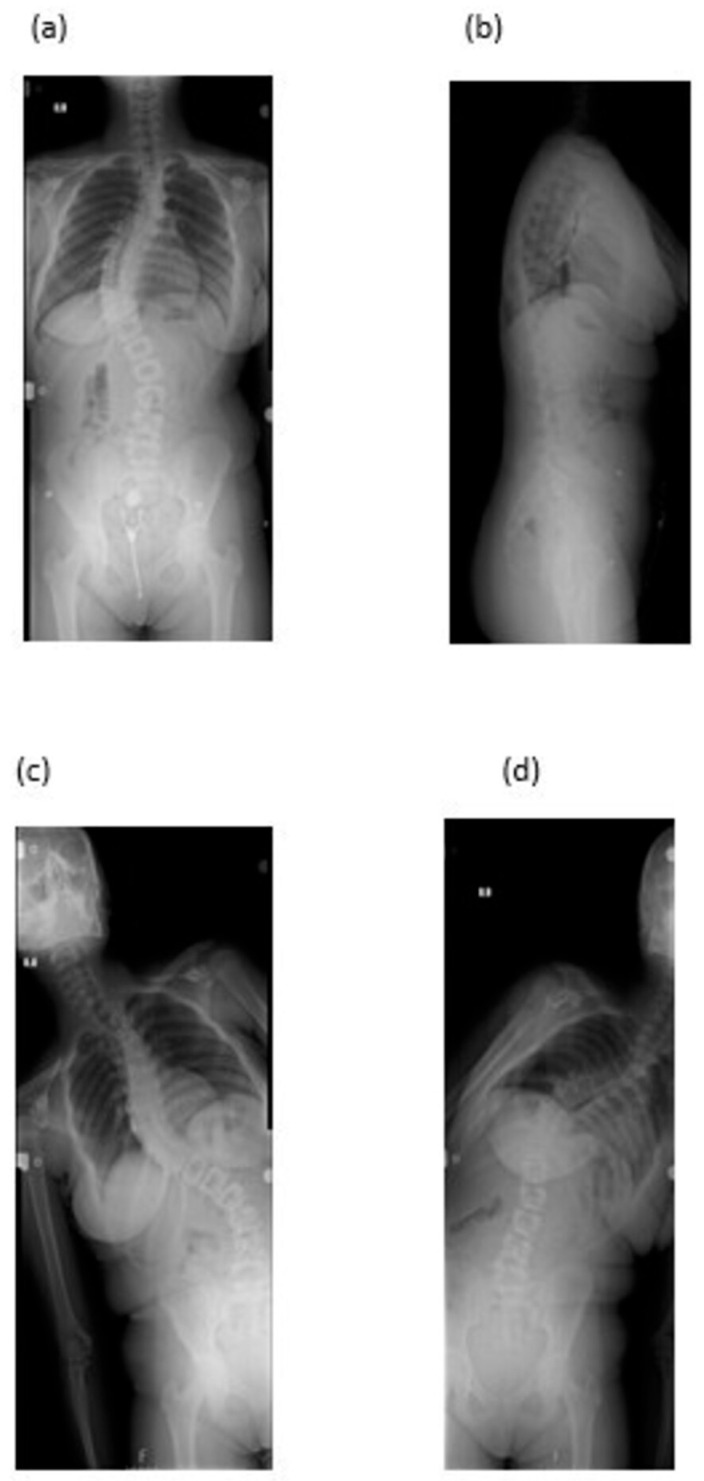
Representative full-length spine radiographs uploaded to the AI systems for analysis: (a) Standing anteroposterior (AP) view; (b) standing lateral view; (c) right bending; and (d) left bending radiographs obtained according to the standardized institutional scoliosis imaging protocol. Images were acquired using a flat-panel digital radiography system (D-EVO Suite II 180, Fujifilm Corporation, Tokyo, Japan) at a 2 m source-to-detector distance and exported in PNG format for AI evaluation.
